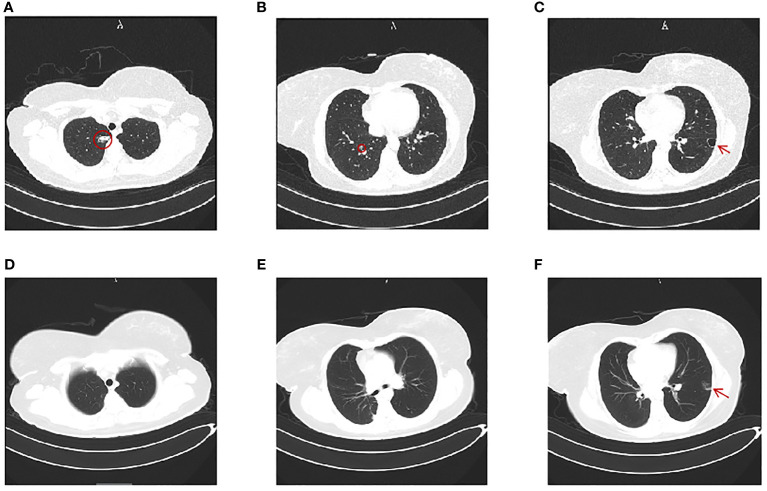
Chest computerized tomography (CT). (A–C) Chest CT image before the first video-assisted thoracoscopic right upper wedge resection and right lower segmentectomy. (A) A mGGN of 24 mm × 14.2 mm in the apical segment of the upper lobe (red circle). (B) A mGGN of 10 mm × 8 mm in the posterior basal segment of the right lung, marked by a red circle. (C) A thin-walled cavity in the posterior segment of the left lower lobe (red arrow). (D–F) Chest CT image 2 months after the first thoracic surgery. (D, E) Postoperative changes of the right lung; no mGGN seen. (F) The mGGN in the posterior segment of the left lower lobe was enlarged at 10 mm × 17 mm and suspected as nodular consolidation (red arrow).
